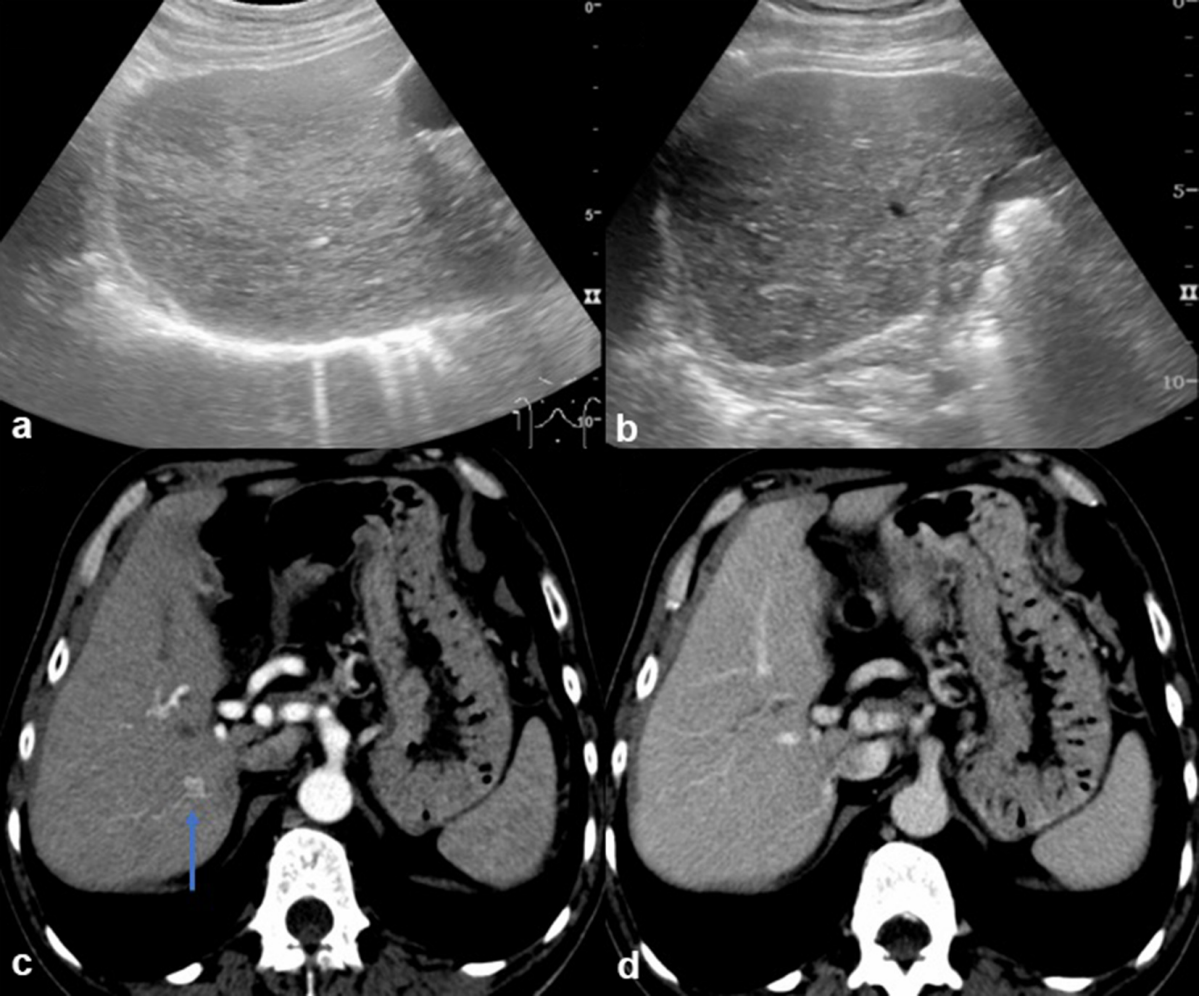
Abdominal ultrasound revealed a normal-sized liver, with a diffusely heterogeneous echostructure suggesting chronic liver disease (a and b). On CT (c and d) the liver is slightly dysmorphic. There is a nodular liver lesion in the right lobe, with CT enhancement pattern in the arterial (c) and portal (d) phases suggesting a benign vascular shunt (arrow in b), which is stable on comparing with multiple previous examinations (not shown).
